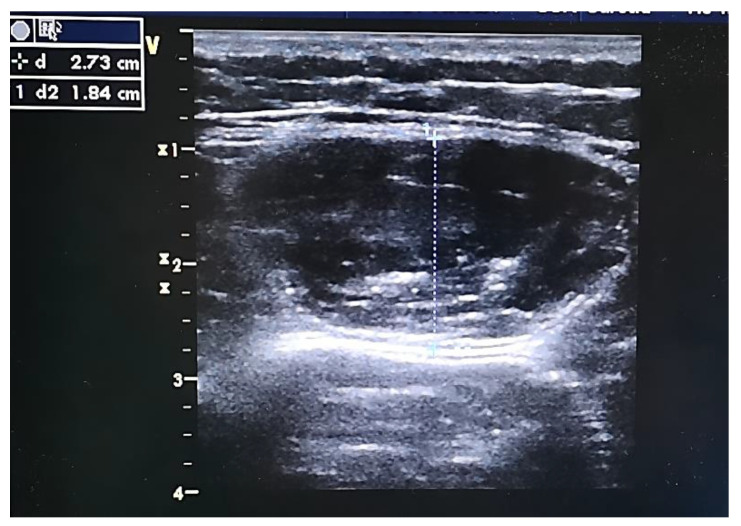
Ultrasonography picture of the RF.
